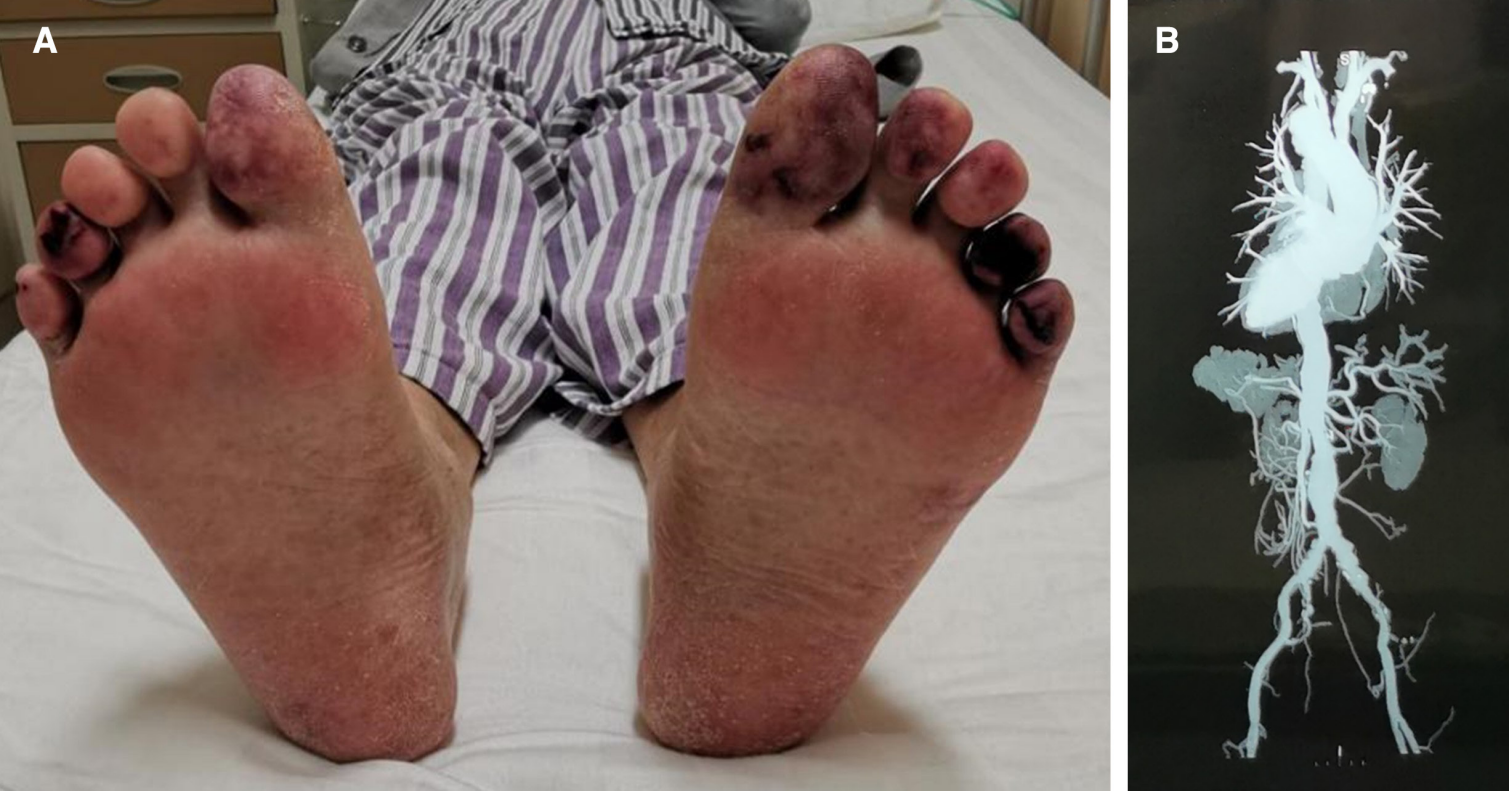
(A) blue toe. (B) The B shows calcified atherosclerotic plaque throughout the visualized thoracoabdominal aorta (arrow) on a three-dimensional computed tomography reconstruction.
